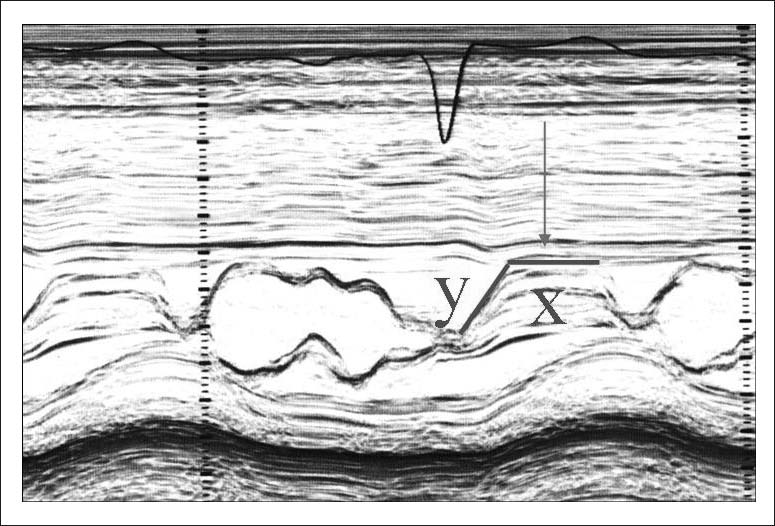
Systolic anterior motion (SAM) of the mitral valve visualized by M-Mode echocardiography. X represent the duration of SAM, whereas Y represent the time elapsed between the beginning of SAM and the SAM-septal contact (see text for more details).
